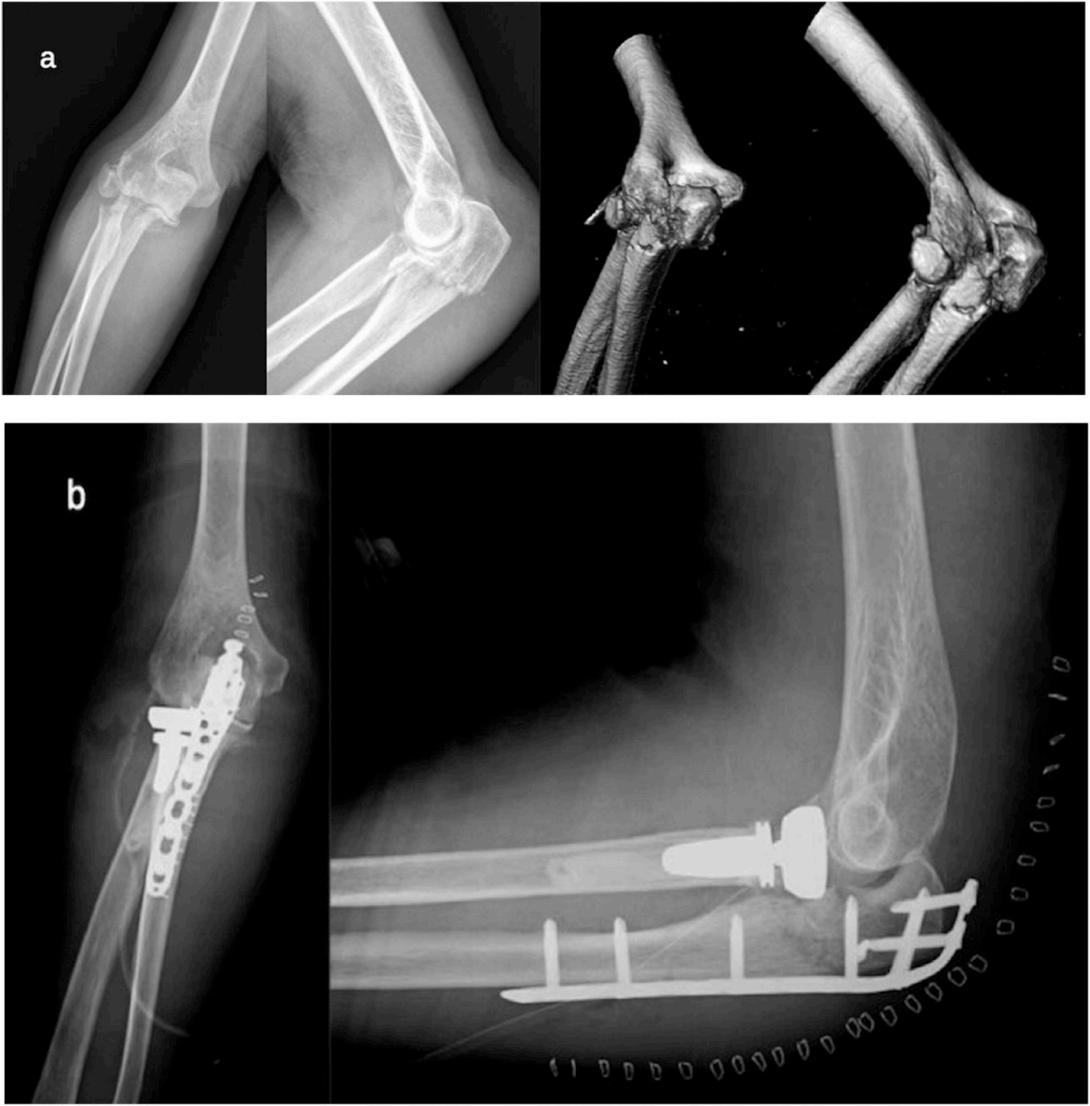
a) Pre-operative radiography and CT scan: simple fracture of the proximal ulna and comminuted fracture of the radial head in osteoporotic bone in a 74- year-old woman. b) Post-operative radiography: global posterior approach and subcutaneous Kocher approach– Pre-contoured locking plate and cemented radial head prosthesis.-*Simple and complex proximal olecranon and/or metadiaphyseal ulnar fracture + fracture – subluxation of radial head + coronoid fracture (large fragment) (*Fig. 3a).
